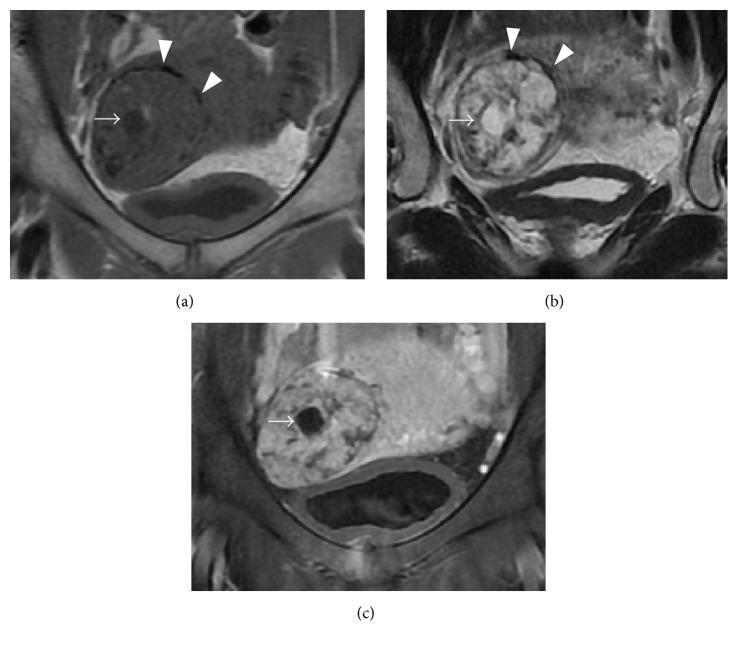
Coronal MRI images. (a) T1-WI shows an isosignal intensity mass in the right cornu. The mass includes a cyst (arrow) and shows low signal intensity. Several flow voids (arrow heads) are observed at the edge of the mass. (b) T2-WI. The mass and cyst (arrow) show high signal intensity. Several flow voids (arrow heads) are observed at the edge of the mass. (c) Gadolinium-enhanced and fat-suppressed T1-WI demonstrates a well-enhanced mass and clear margins. Arrow indicates the cyst.
